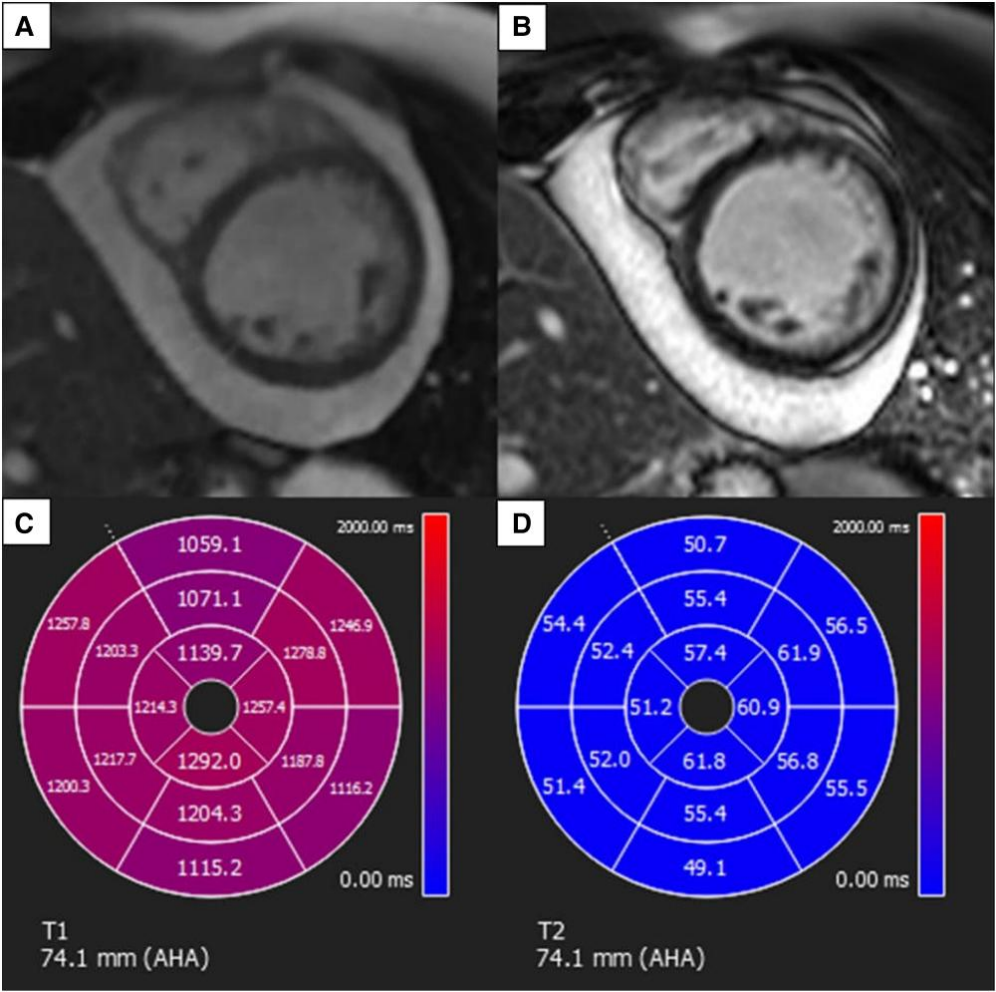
Cardiac magnetic resonance images. Compiled cardiac magnetic resonance images at the mid left ventricle. (*A*) Cine short-axis image. (*B*) Corresponding late gadolinium enhancement short-axis image shows subepicardial hyperenhancement at the lateral segment. (*C*) T_1_ polar map shows elevated values at all segments, in keeping with diffuse interstitial fibrosis. (*D*) T_2_ polar map shows elevated values at all the lateral segments as well as the mid to apical anterior and inferior segments, sparing the septal segments. Findings of subepicardial hyperenhancement and elevated T_2_ polar map values at the lateral segments are in keeping with features of acute myocarditis.
